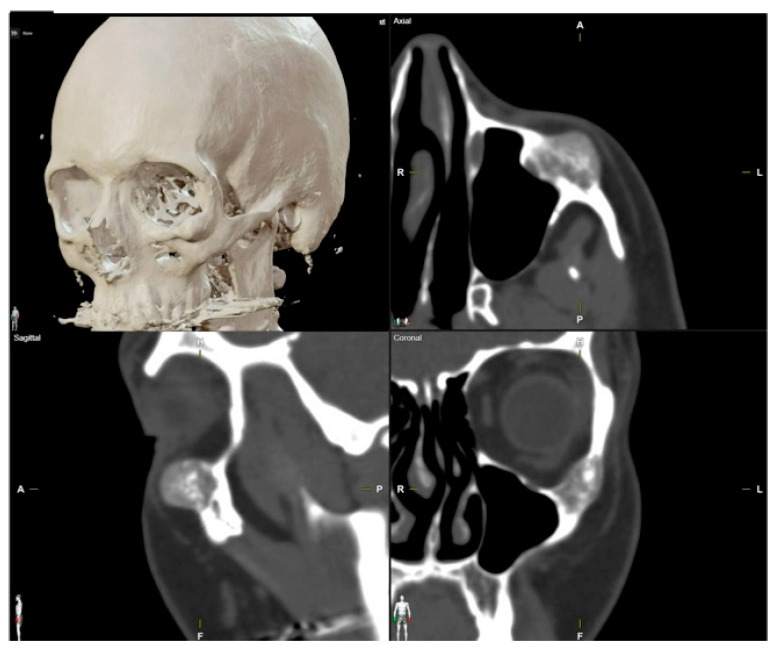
Preoperative CT scan. Three-dimensional, axial, sagittal and coronal views can be appreciated. There are a mixed radiolucent lesion and an expansive lytic defect affecting the left infraorbital rim, zygoma, external zone of the orbital floor and inferior part of the external wall.
